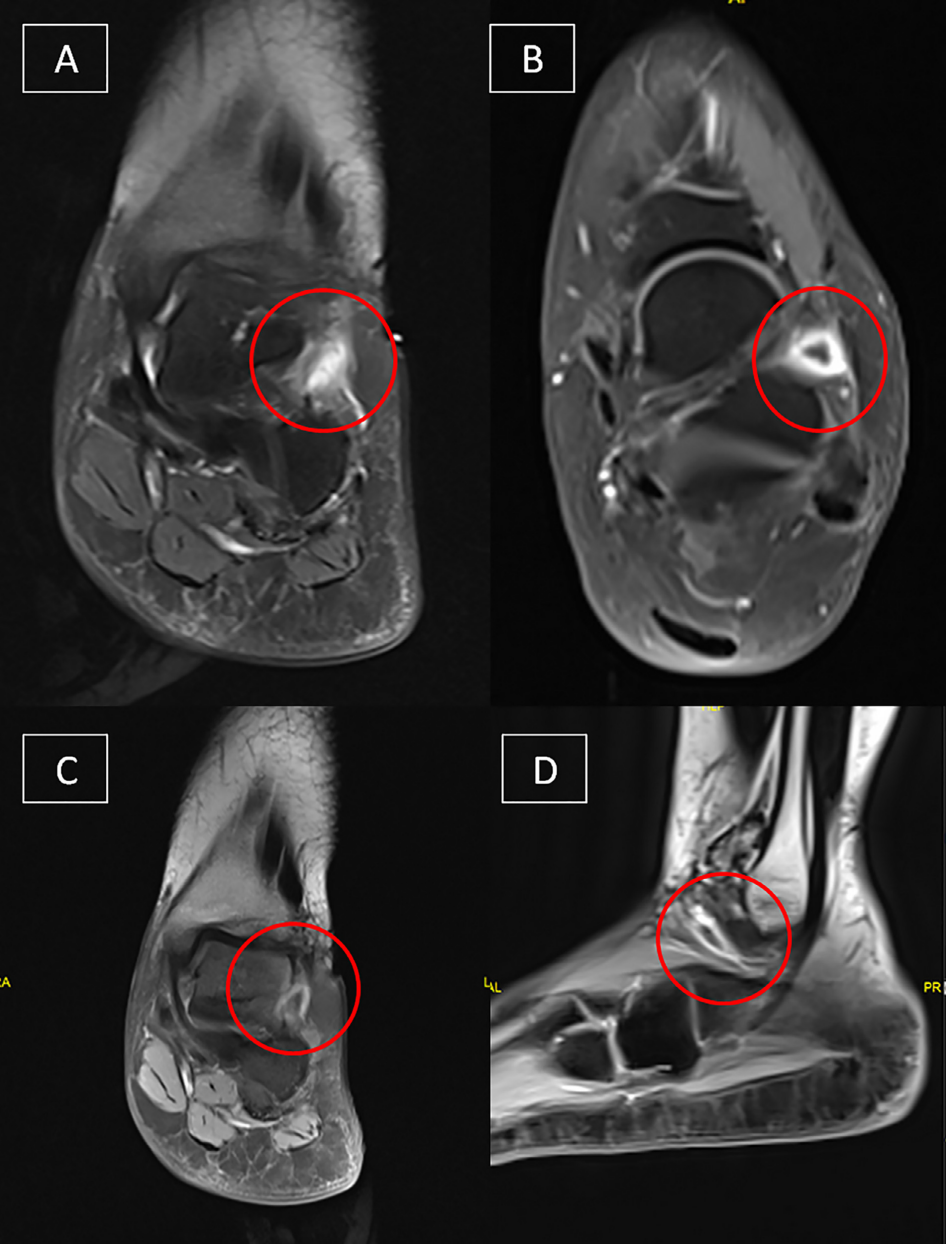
(A) axial and (B) coronal T1 FAT SAT with contrast demonstrating a well-defined ovoid cystic lesion (red circles) lining the inferior extensor retinaculum as it passes over the extensor digitorum longus tendon.(C) Coronal and (D) Sagittal images show the same findings (red circles). The precise anatomical locations of the latter, as well as the MRI findings, are consistent with a diagnosis of Gruberi bursitis. The bursa, on the other hand, is reaching the subtalar joint space but there is no definitive communication. Additionally, there is no associated ligamentous injury or osteochondral lesion, and the bone marrow signal intensity is normal.
